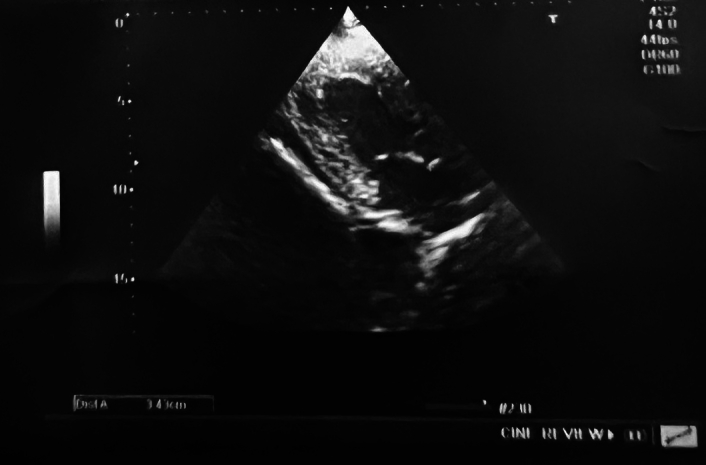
Echocardiography with a parasternal long axis view showing thickening of the left ventricular wall suggestive of left ventricular hypertrophy.
